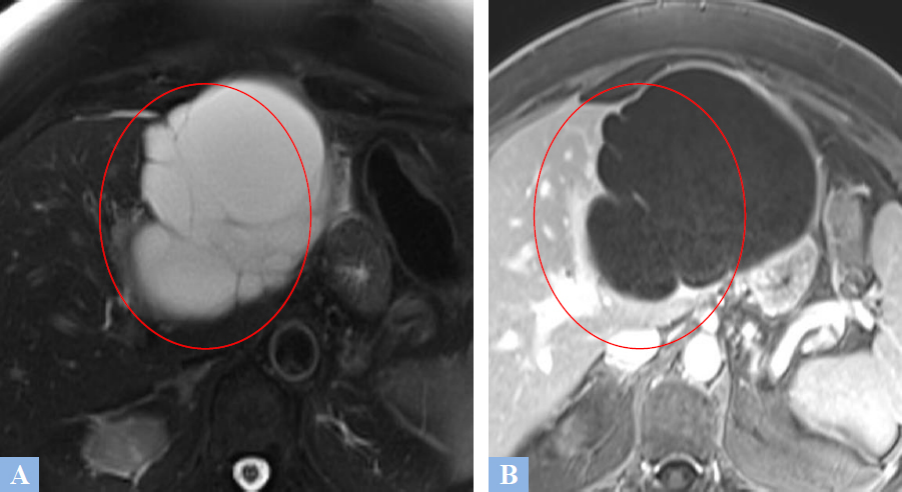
Multiloculated biliary cyst mistakenly diagnosed as a mucinous cystadenoma in a 67-year-old female patient. A. T2-weighted fat-suppressed MR image in the axial plane. Lobulated cystic lesion containing intracystic septations appearing as T2 hypointense. B. T1-weighted fat-suppressed MR image in the axial plane after gadolinium administration at the portal phase of enhancement showing mild enhancement of the intracystic septations (red circle).
